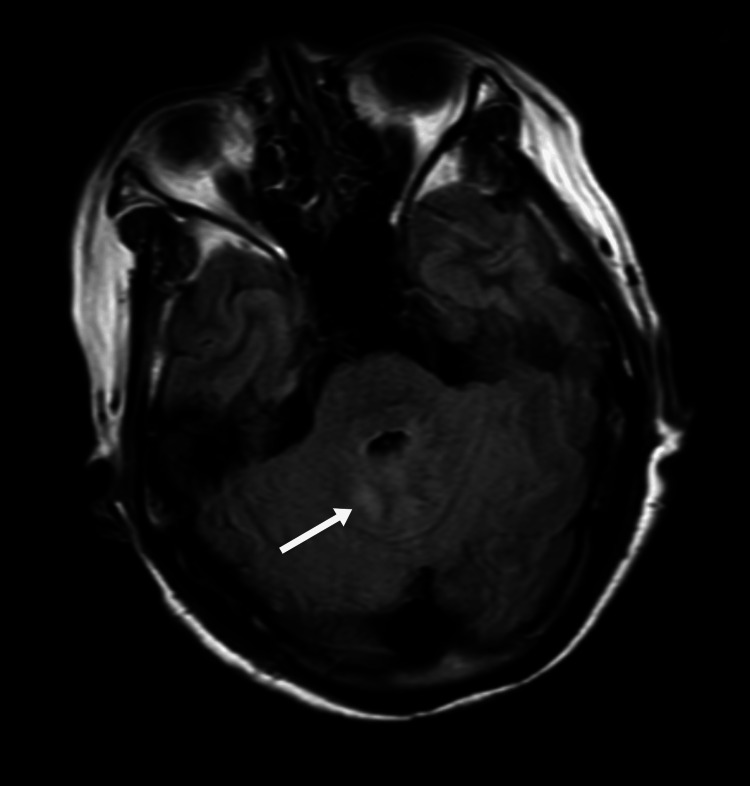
Axial FLAIR MRI of the cerebellar dentate nucleus before treatment As indicated by the white arrow, there is an abnormally high signal in the bilateral symmetric dentate nuclei of the cerebellum FLAIR: fluid-attenuated inversion recovery; MRI: magnetic resonance imaging
